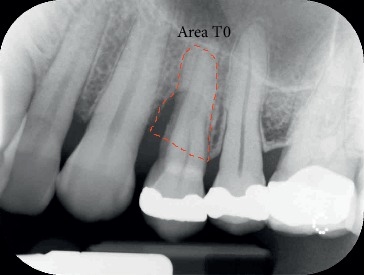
Tooth 2.4: X-ray at T0: the area selected (red contour) represents the part of the root inside both the bone and the defect.
